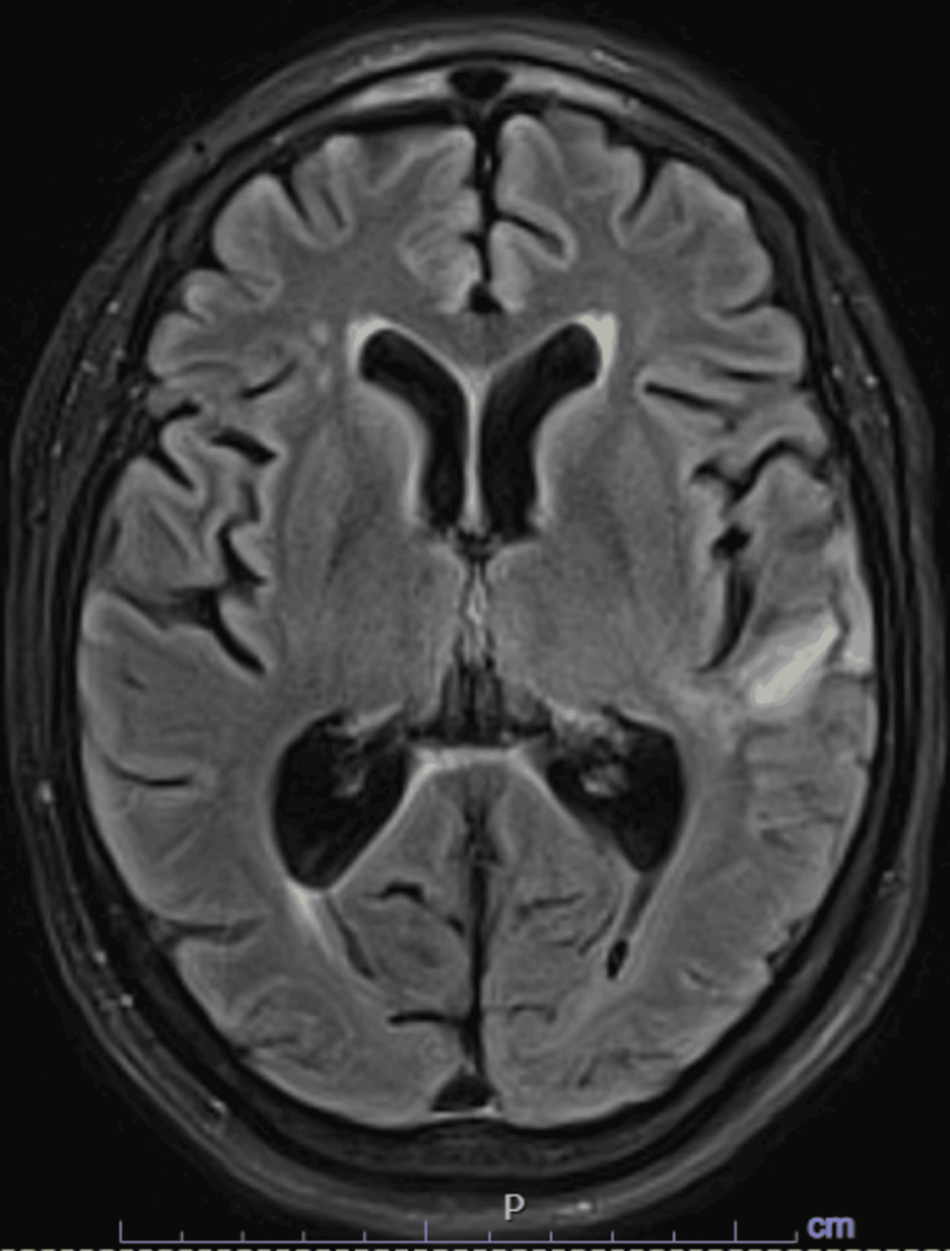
Follow-up MRI after three months showing significant improvement in left temporal lobe edema is noticed
